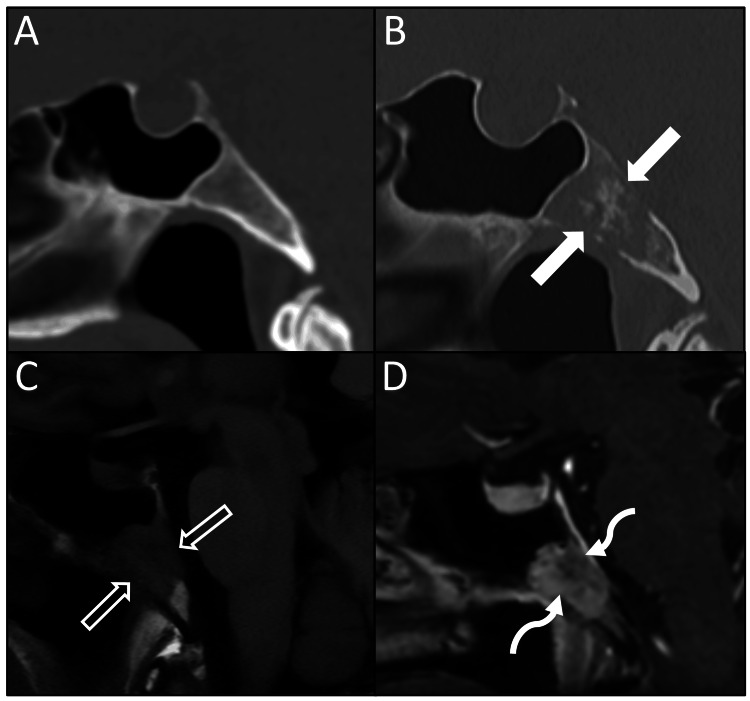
Progressive development of clival lesion in a patient with known vestibular schwannoma Surveillance CT for known vestibular schwannoma demonstrates normal clivus on sagittal reconstruction (A). Four years later, surveillance CT demonstrates an expansile lytic lesion (B, solid arrows) at the clivus on sagittal reconstruction. Follow-up sagittal MRI images demonstrate the mass to be marrow-replacing with T1 hypointensity (C, open arrows) and heterogeneous enhancement (D, curved arrows).
